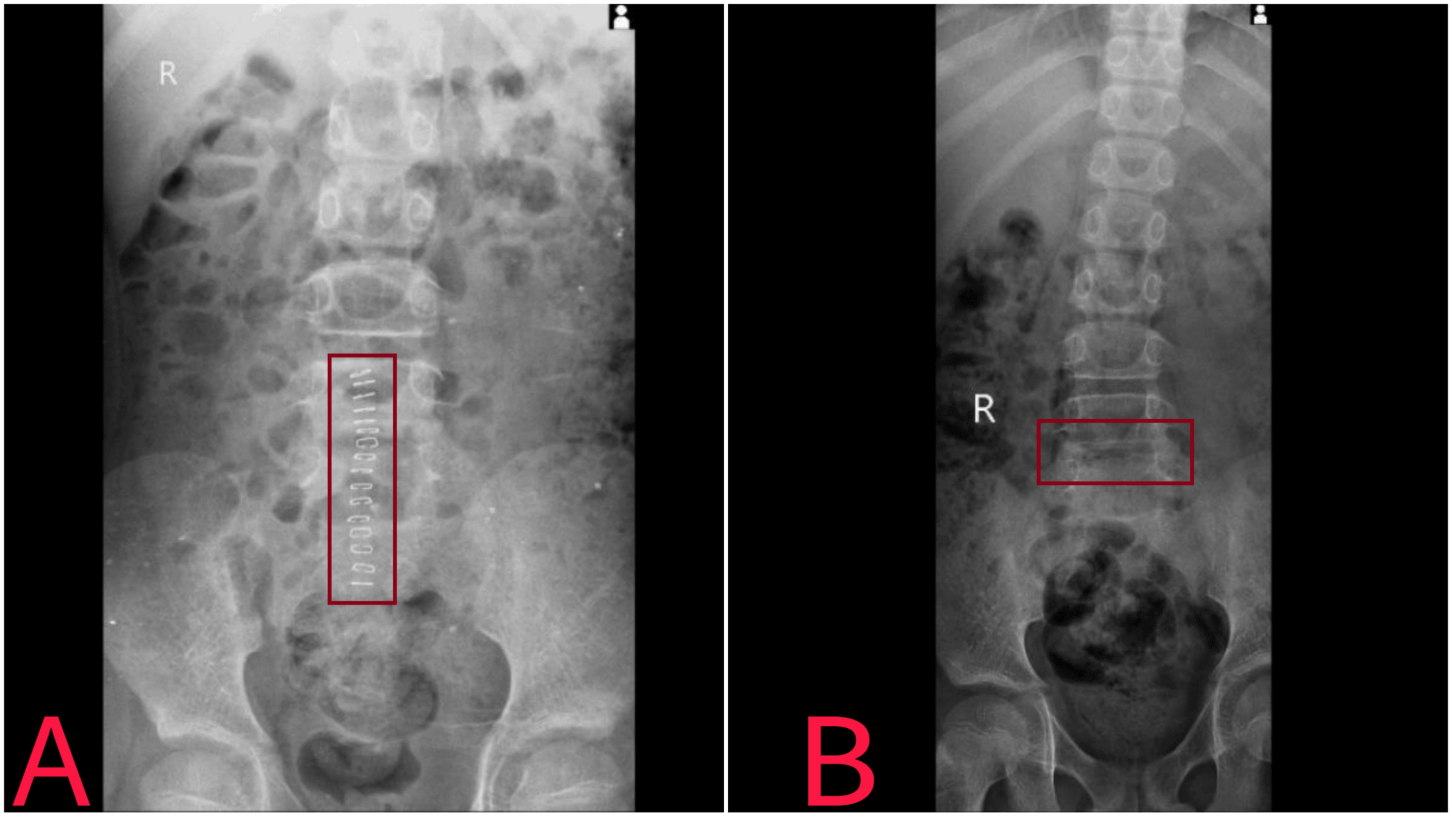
X-ray of the spine Figure A: Post-X-ray shows that posterior decompression and spinal fusion have been performed. Figure B: Pre-X-ray reveals reduced vertebral body height at the L5 and S1 levels, sclerotic changes, degenerative changes in the intervertebral space, and a decrease in two interspinous processes.
